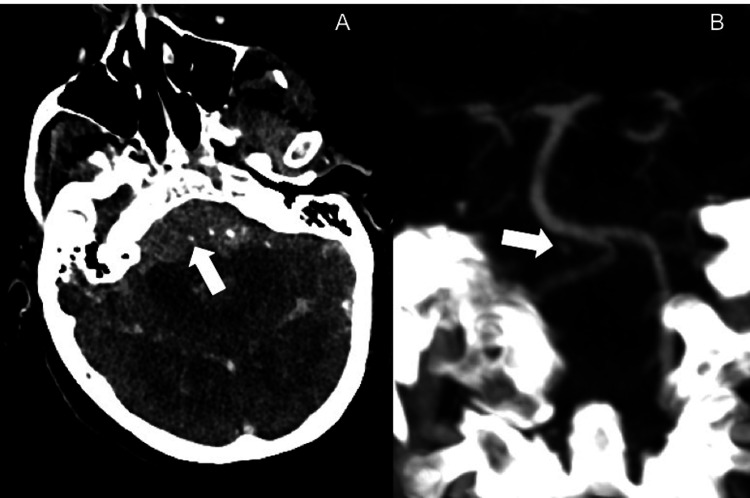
Ruptured basilar perforator aneurysm on CT. Axial (A) and coronal (B) CT angiogram images showing focal contrast pooling adjacent to the proximal basilar artery.
